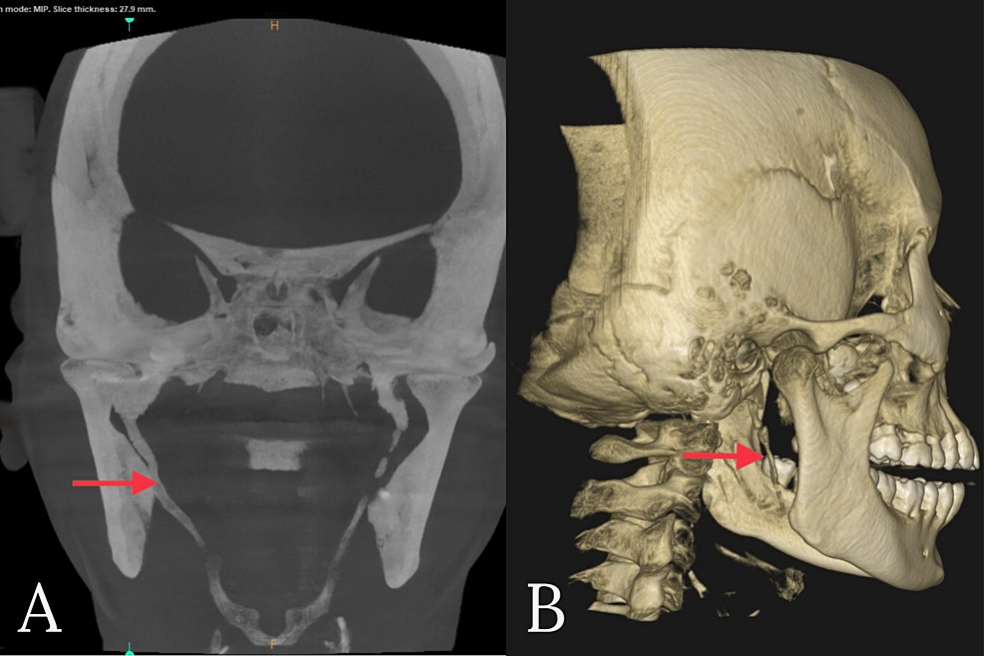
Pre-operative CBCT The arrow mark represents the elongation of the styloid process showing S curvature, the downward and forward directions in a coronal view of CBCT (A), and the 3-dimensional view of CBCT (B)
